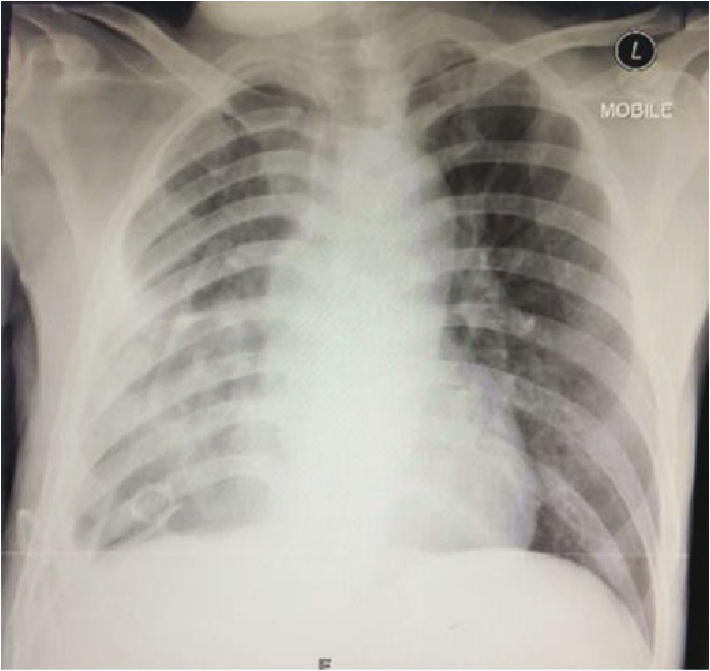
Chest radiography showed consolidative changes with mild to moderate pleural effusion on the right side.
